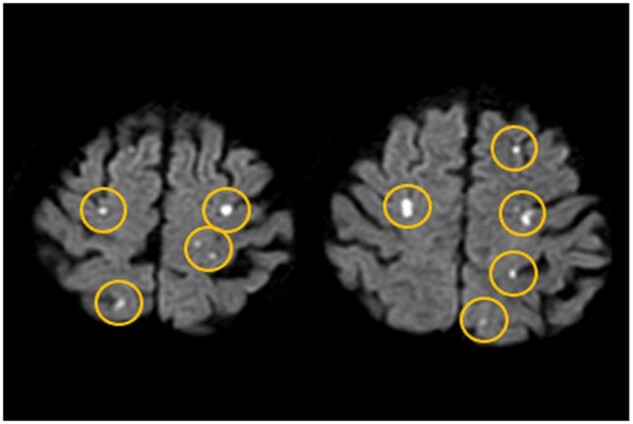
Impressive diffusion-weighted magnetic resonance imaging of multiple asymptomatic cerebral infarction after thoracic endovascular aortic repair.
